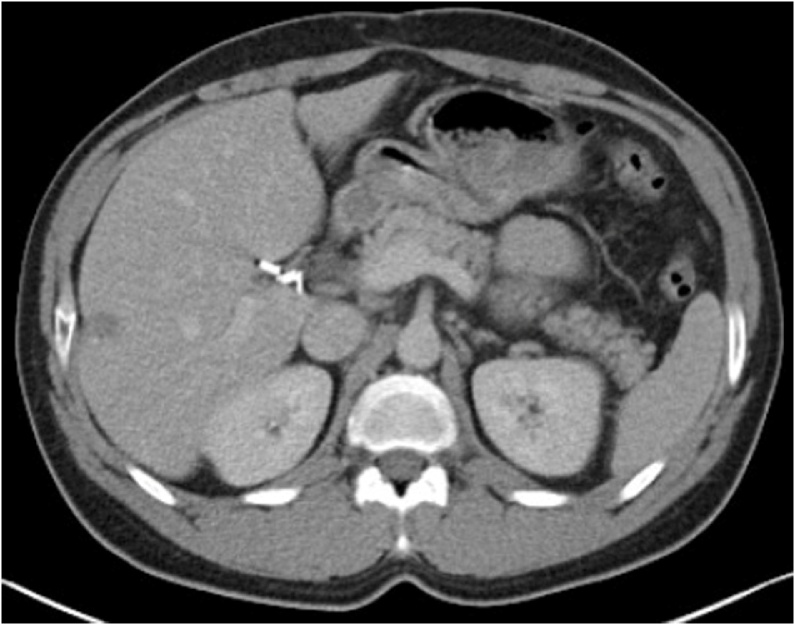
Abdominal computed tomography image demonstrating liver metastasis after chemotherapy.
